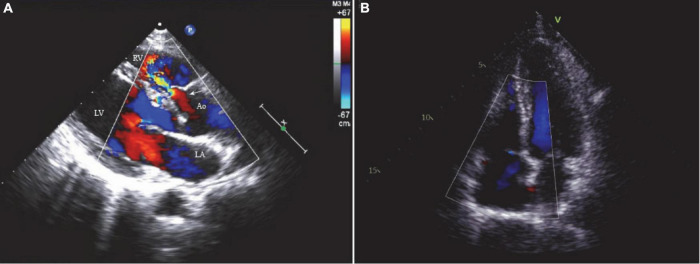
(A) The two-dimensional echocardiogram showed a 10.6 mm × 9.6 mm ruptured sinus of Valsalva aneurysm (right coronary sinus) and a 5.0 mm aortocardiac shunt (*white arrow*). (B) No residual shunt or valve regurgitation noted on the postoperative echocardiogram. LA, left atrium; LV, left ventricle; RV, right ventricle; Ao, aorta.
